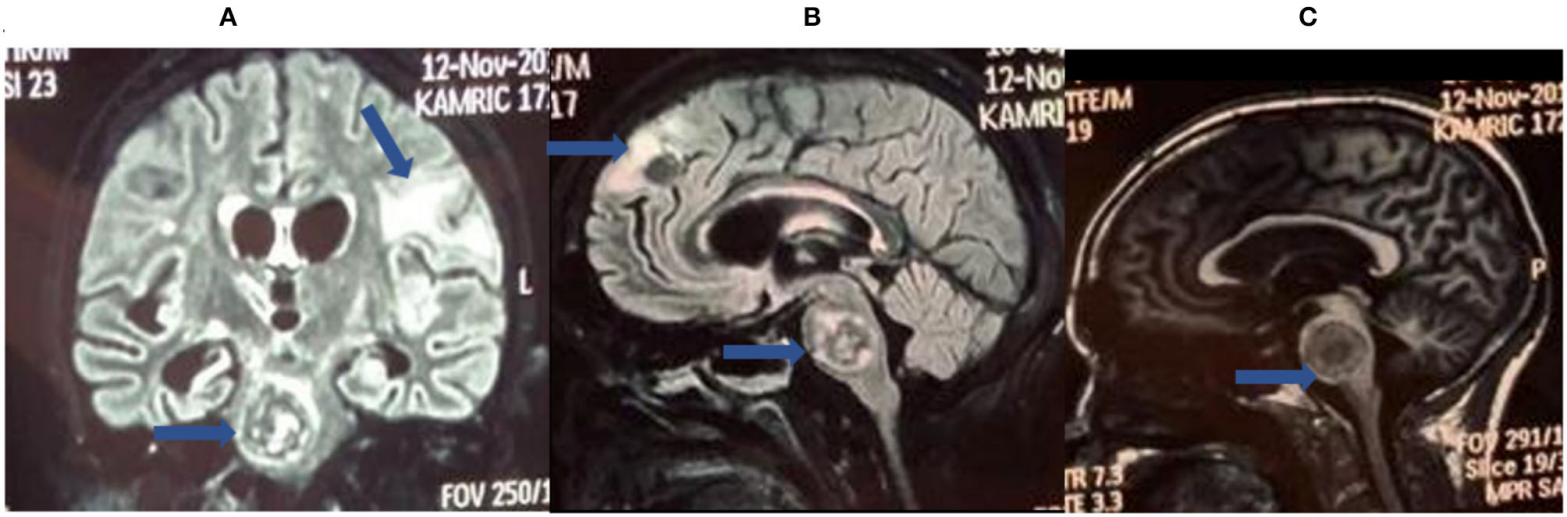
Brain magnetic resonance imaging (MRI) for tuberculous meningitis (TBM) diagnosis. These are selected images from a brain MRI obtained from a patient with definite TBM showing cerebral space occupying lesions (tuberculomas) in the brainstem (A–C) and frontal lobe along with white matter ischemic changes (B).
